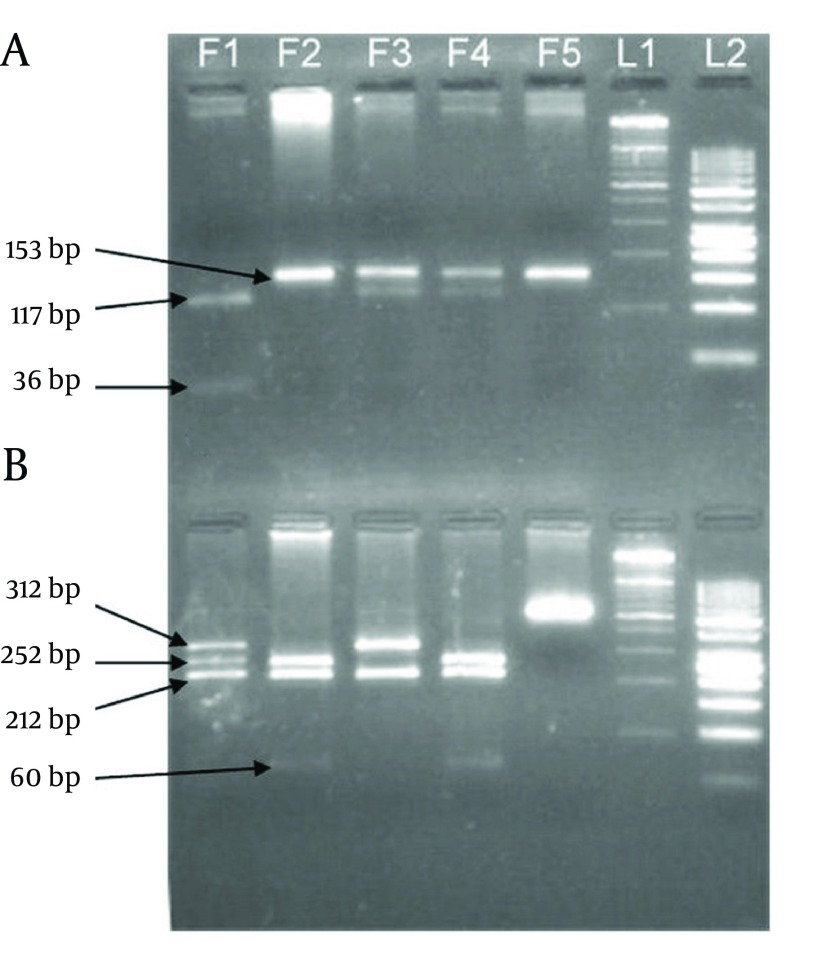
Products of Enzymatic Digestion and Their Fragments Size on Agarose Gel Electrophoresis A) Eco81I fragments (-509C/T polymorphism), B) BglI fragments (+915G/C polymorphism), F1) CC genotype of -509C/T and GC genotype of +915G/C, F2) TT genotype of -509C/T and GG genotype of +915G/C, F3) CT genotype of -509C/T and CC genotype of +915G/C, F4) Positive control, F5) Negative control (PCR product), L1) 100 bp DNA ladder, L2) 50 bp DNA ladder.
